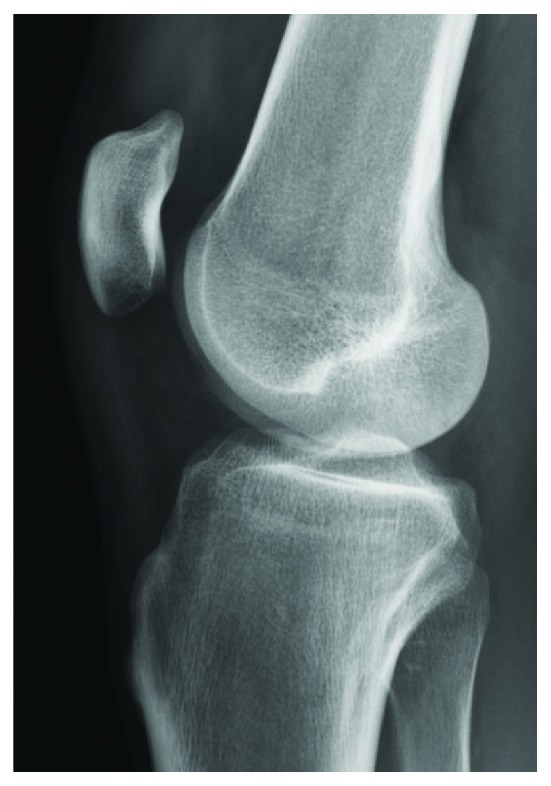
Preoperative X-ray of the contralateral knee to measure the Caton-Deschamps index and patellar height.
